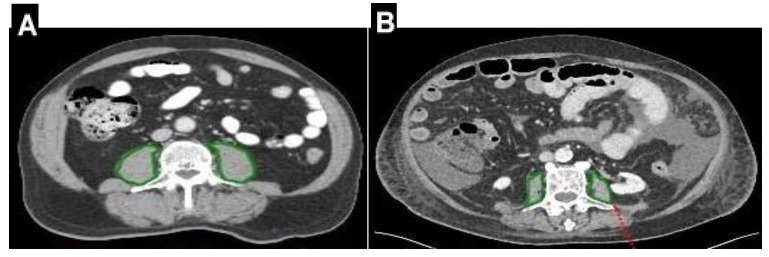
Axial CT images at the L3 vertebral level. The green line shows the outline of the psoas muscle. Measurements were performed in a semi-automated fashion with manual outlining of psoas muscle borders. Two pictures of axial CT images in two different patients are shown: (A) a patient with mild pancreatitis and PMA 28 cm^2^ and (B) a patient with PMA 11 cm^2^ and marked ascites.
